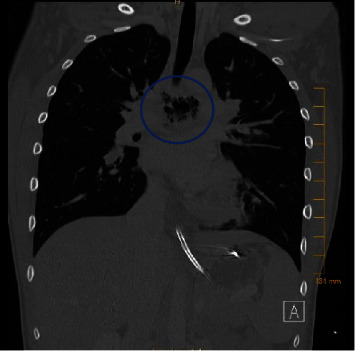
Chest computed tomography revealing a mediastinal abscess.
